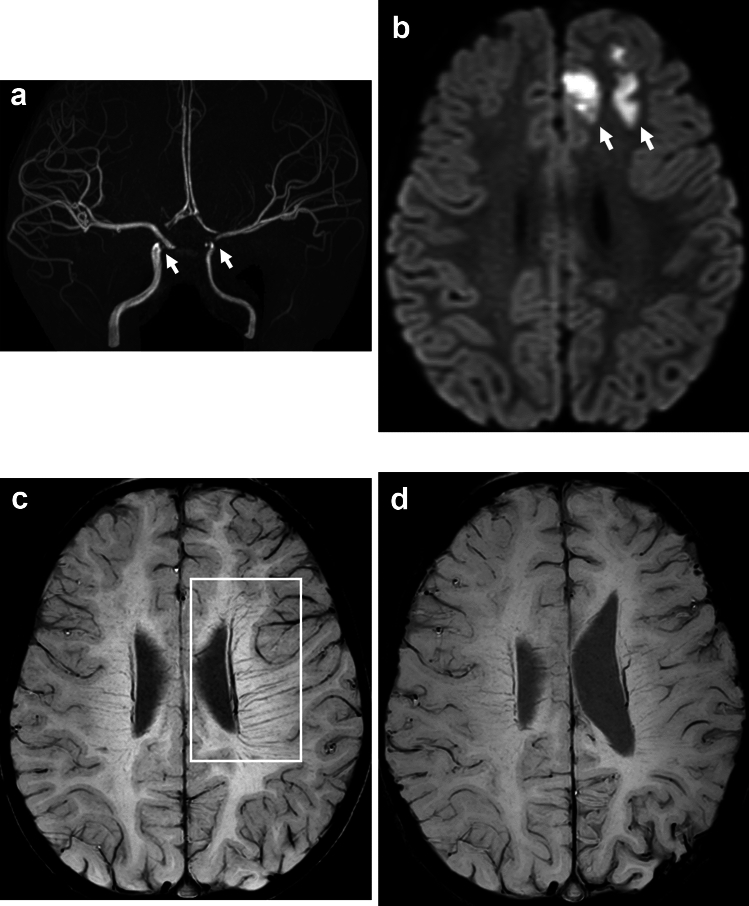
A 2-year-old boy was admitted to our hospital with right-sided hemiparesis after crying. MR angiography shows bilateral internal carotid artery stenosis 2 days after onset (a, arrows). Diffusion-weighted imaging shows acute infarct in the left frontal lobe (b, arrows). SWI shows brush sign on the left side (c, square). The patient was subsequently diagnosed with moyamoya disease based on angiographic findings (not shown) and underwent left-sided bypass surgery. SWI shows disappearance of the brush sign on a follow-up MRI 4 years after onset (d)
